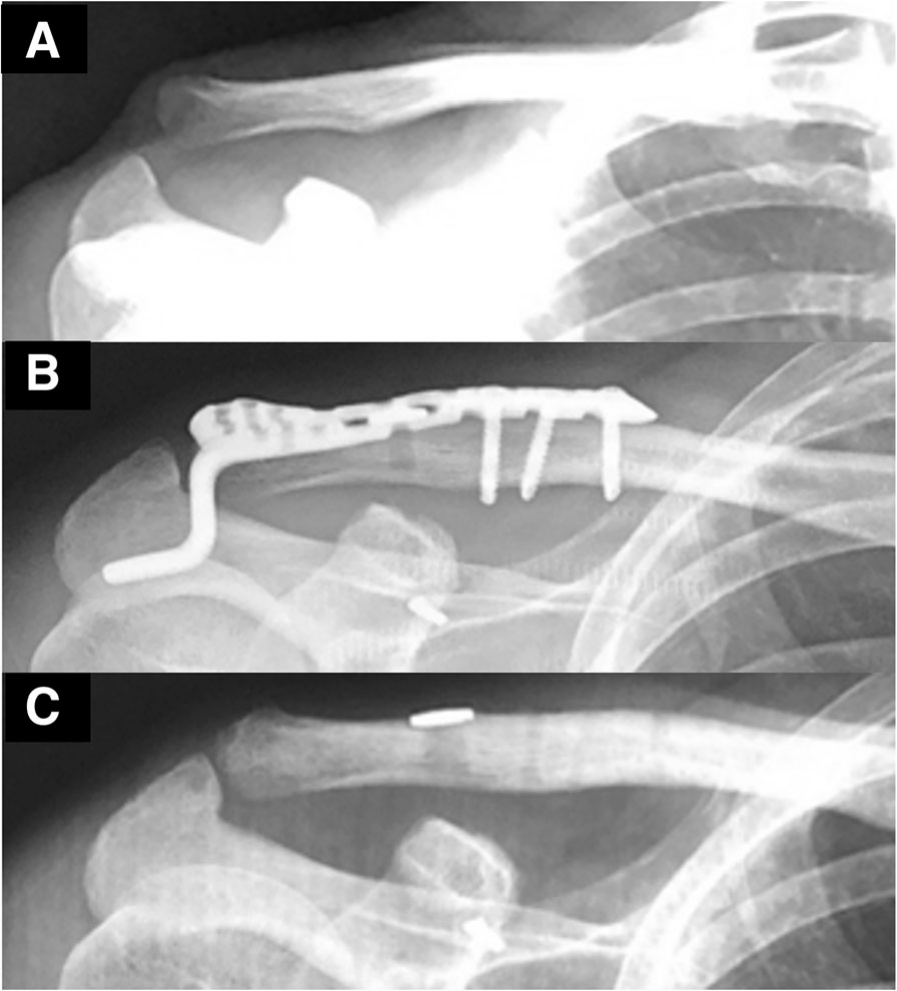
First injury and its treatment. X-ray radiography image at the initial injury demonstrated right acromioclavicular joint (ACJ) dislocation (a), and the patient underwent ACJ reconstruction using a suture-button and a hook plate seven months ago (b). The hook plate was removed four months ago (c)
